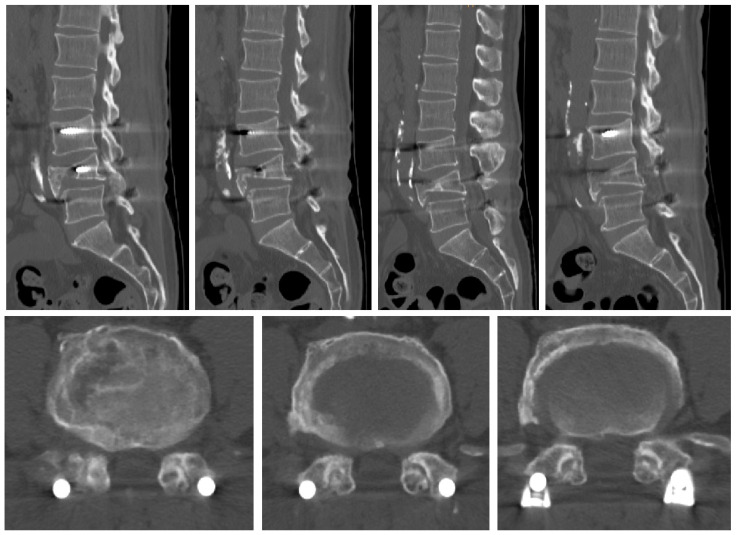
CT imaging showed central stenosis and bilateral neural foraminal stenosis at L4/L5 due to bone overgrowth.
